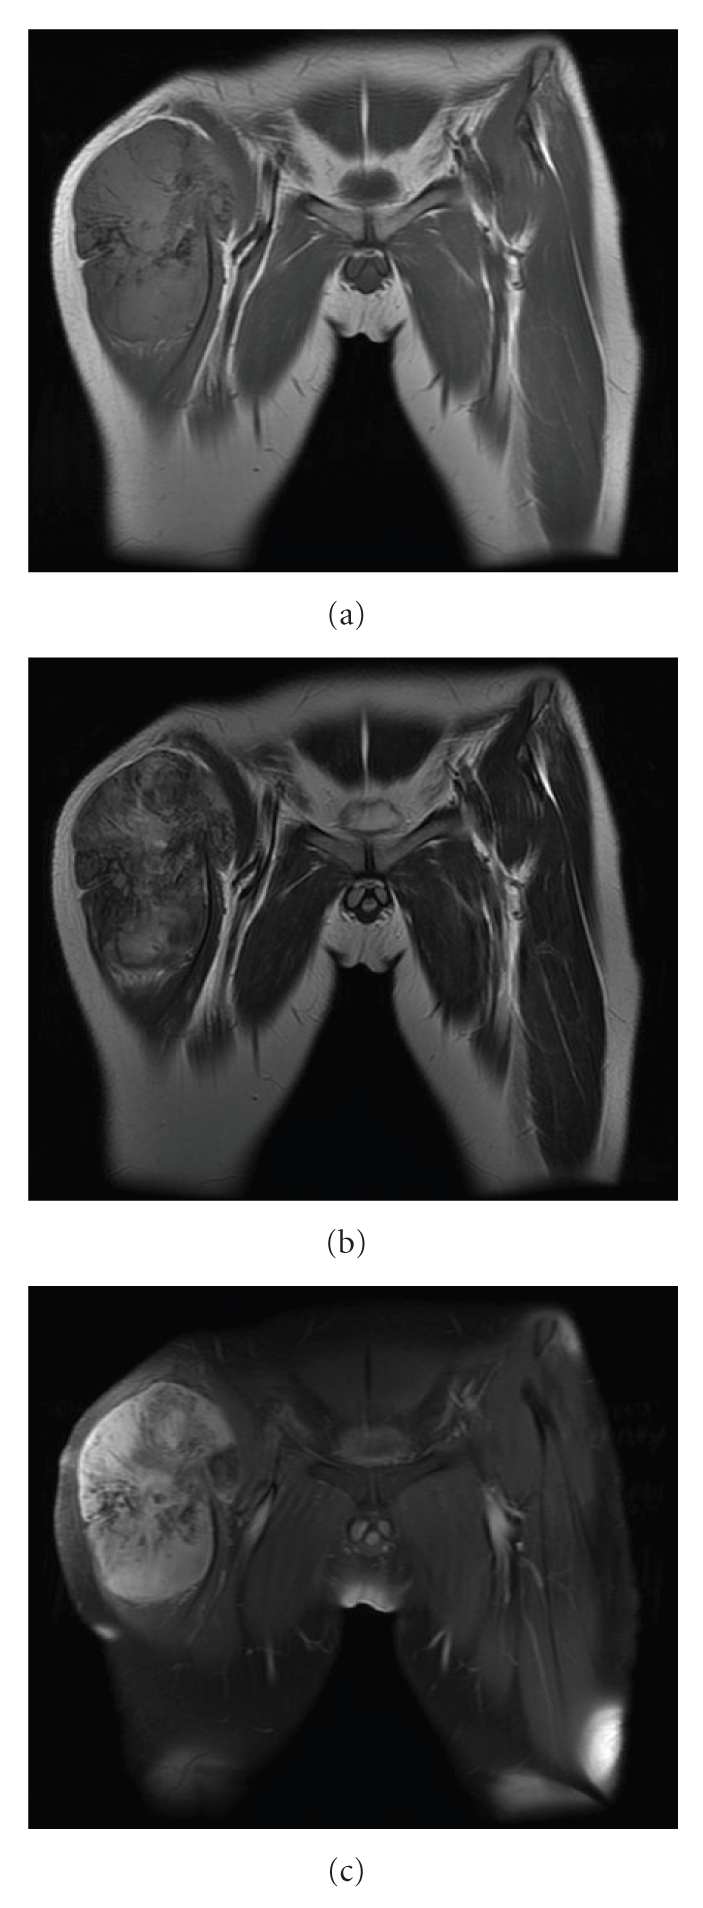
Magnetic resonance imaging of the tumor. (a) T1-weighed MRI. The mass showed mixed low and iso-intensity signals. (b) T2-weighed MRI. The mass showed mixed high and iso-intensity signals. (c) The tumor was enhanced remarkably by gadolinium.
